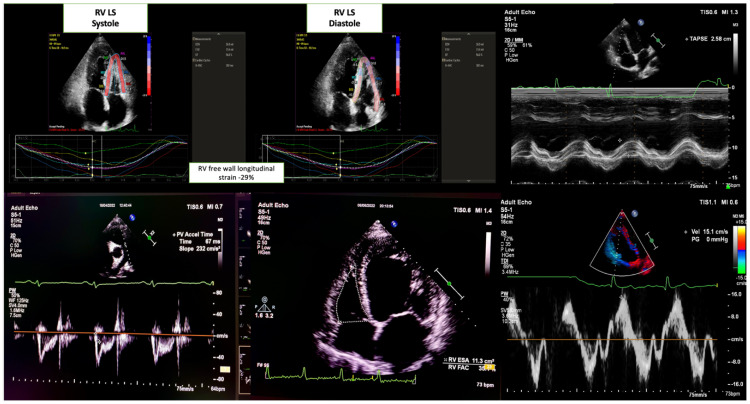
Evaluation of Right ventricular function in patients with acute decompensated heart failure using speckle tracking and other parameters echocardiography.
